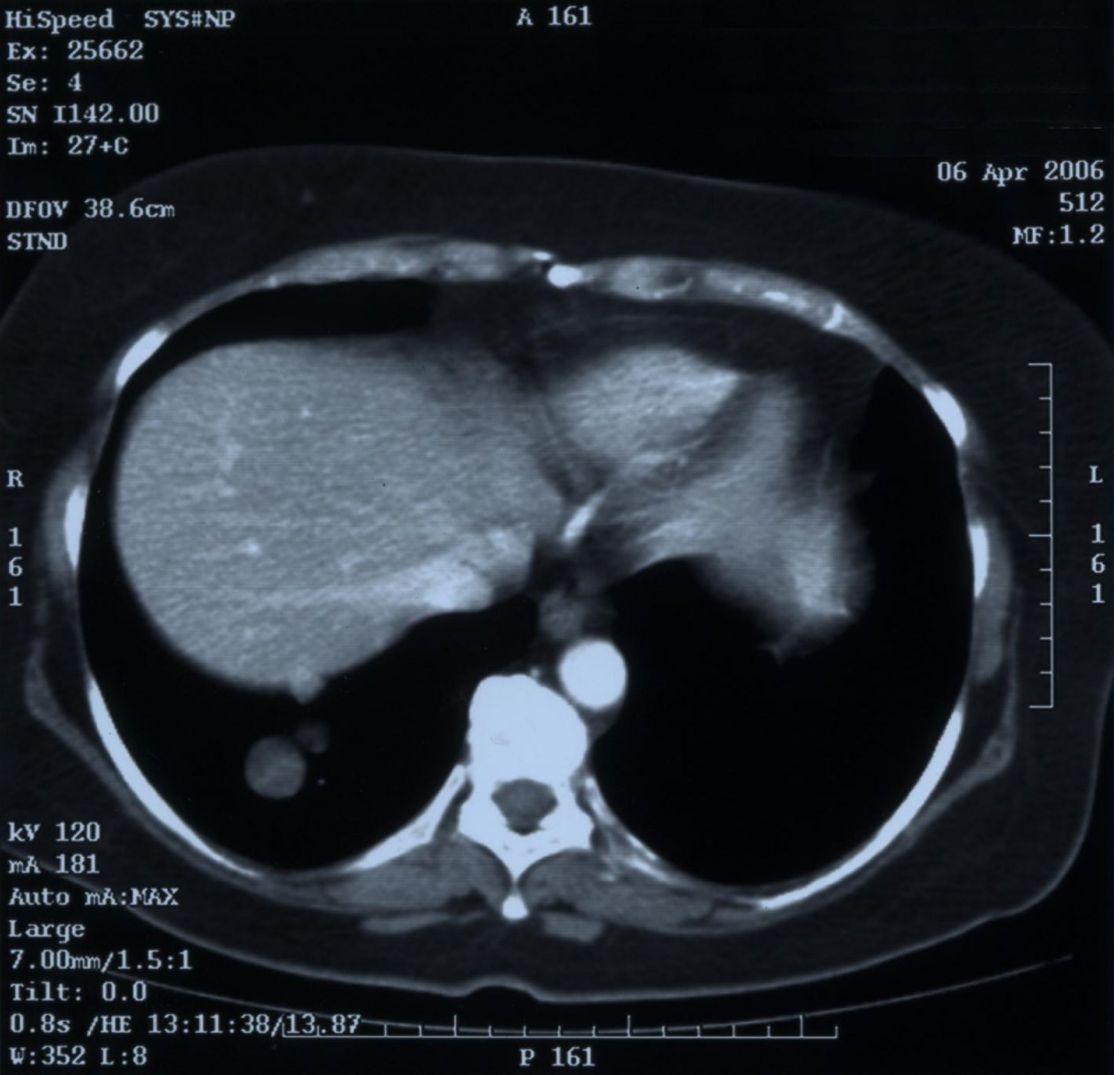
Computed tomography scan of the thorax showing single nodular mass in the right lung of about 4 cm in diameter, evident 1 year after the pelvic recurrence resection.
